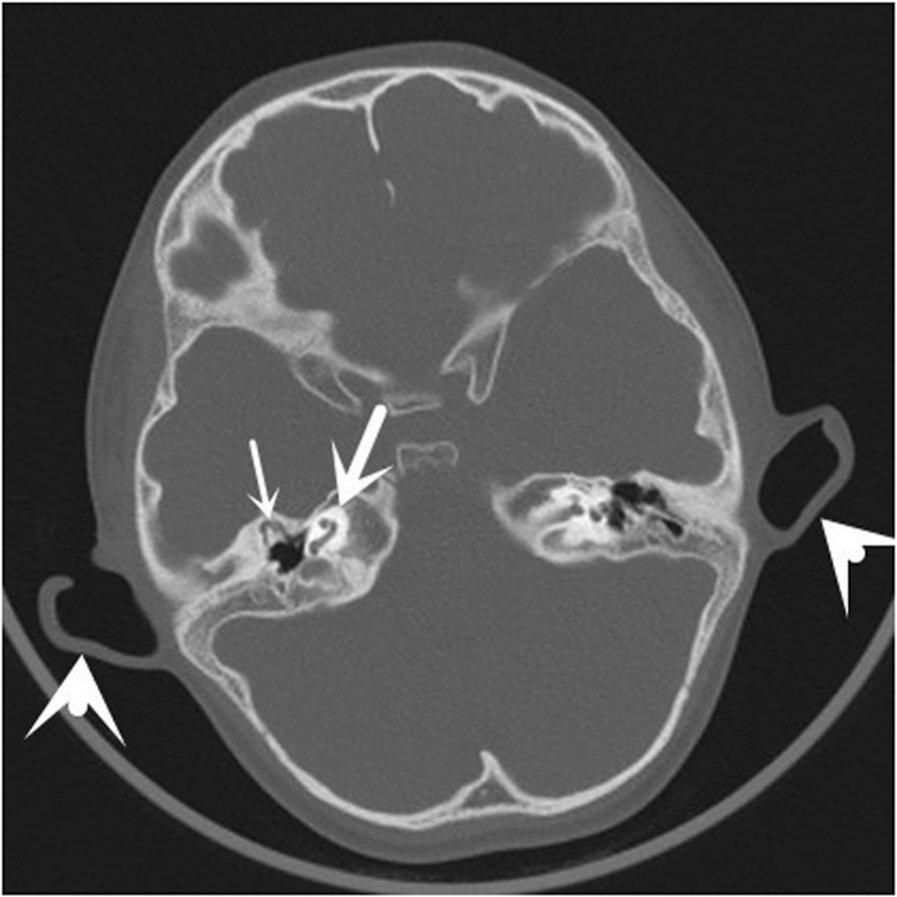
Axial computed tomographic images of the temporal bone from subject III:5. The small arrow indicates a malformed middle ear, including anteriorly displaced abnormal ossicles and hypoplastic mastoid cells. The middle arrow indicates malformed cochlea. The large arrow indicates microtia
